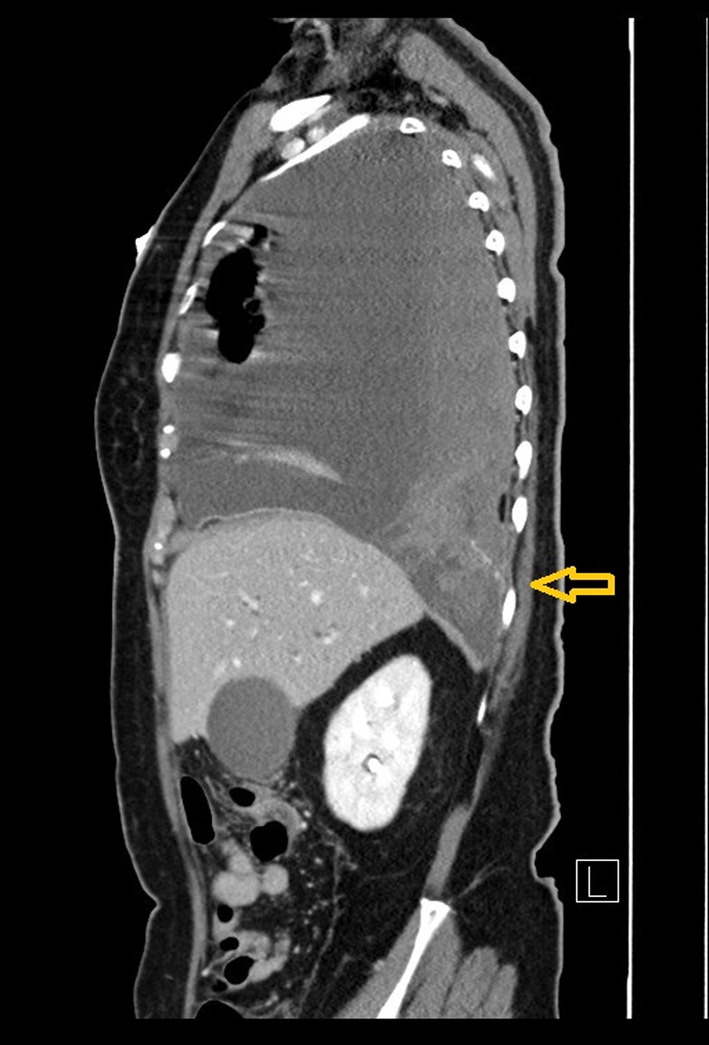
Sagittal view of computed tomography showed extravasations of contrast medium from the right 10th intercostal artery (arrow)
